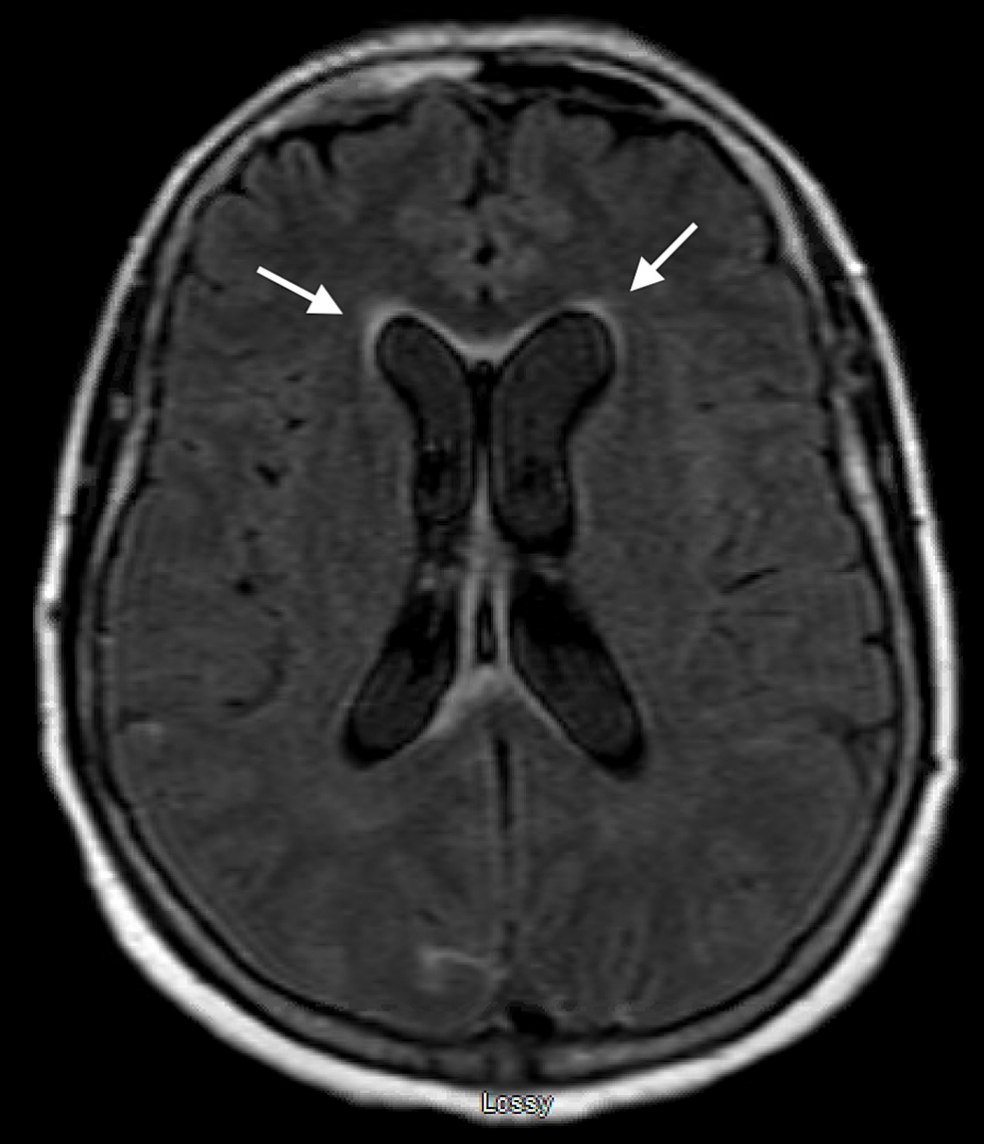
MRI brain FLAIR sequence demonstrating ventriculomegaly in the bilateral lateral ventricles with associated transependymal edema. MRI, magnetic resonance imaging; FLAIR, fluid-attenuated inversion recovery.
